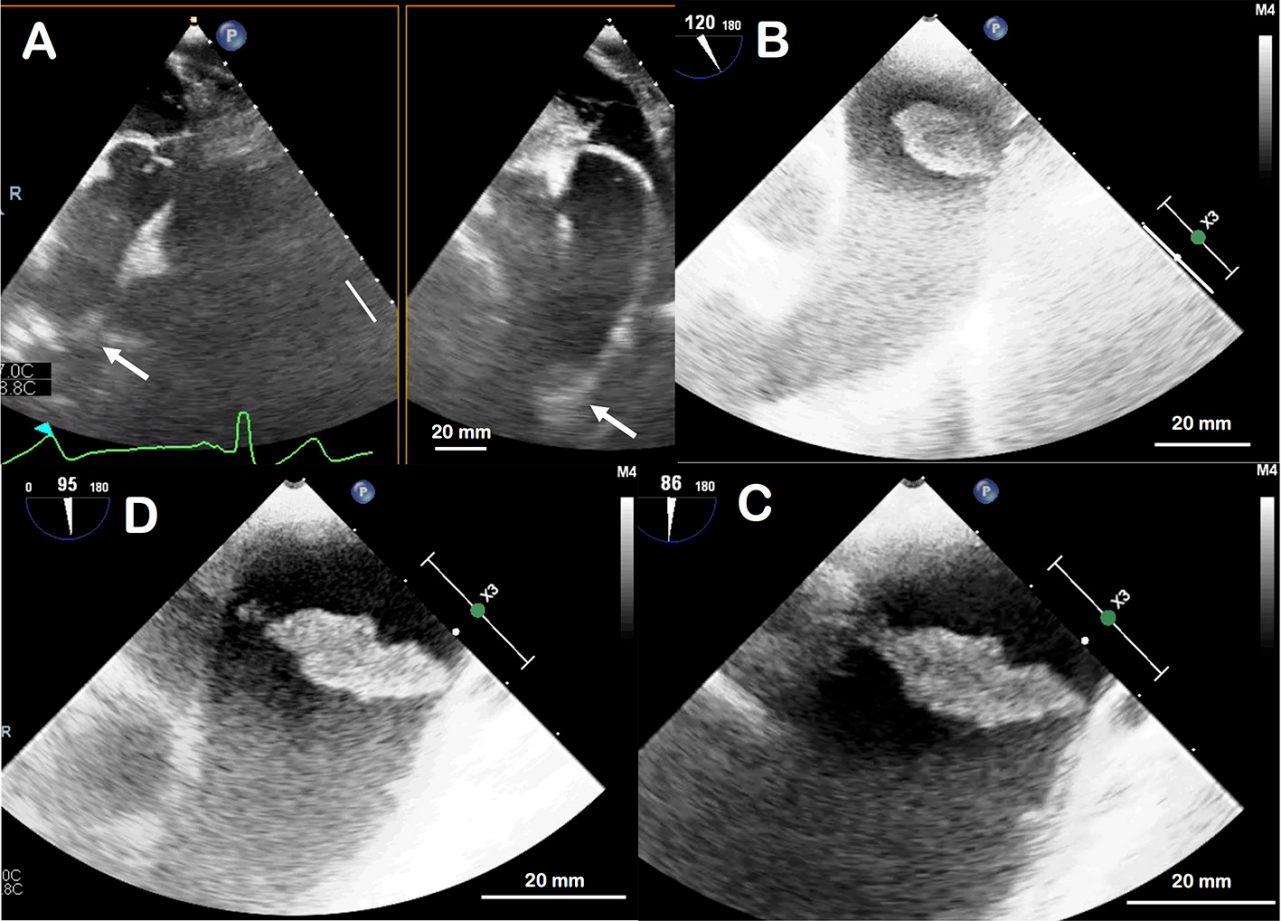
Transoesophageal ultrasound appearance of the thrombus in the aortic arch. Not visible on the initial part of the ascending aorta, it appears only in deep transgastric view by aligning the thoracic aorta (A); white arrow indicates the thrombus. It is located 6 cm far from the aortic valve. The thrombus is then well-visualized by removing the ultrasound probe and aligning the aortic arch (B). By zooming in on the thombus, it appears as a large, mobile and irregular thrombus, attached to a small base at the upper part of the aortic arch (C,D).
